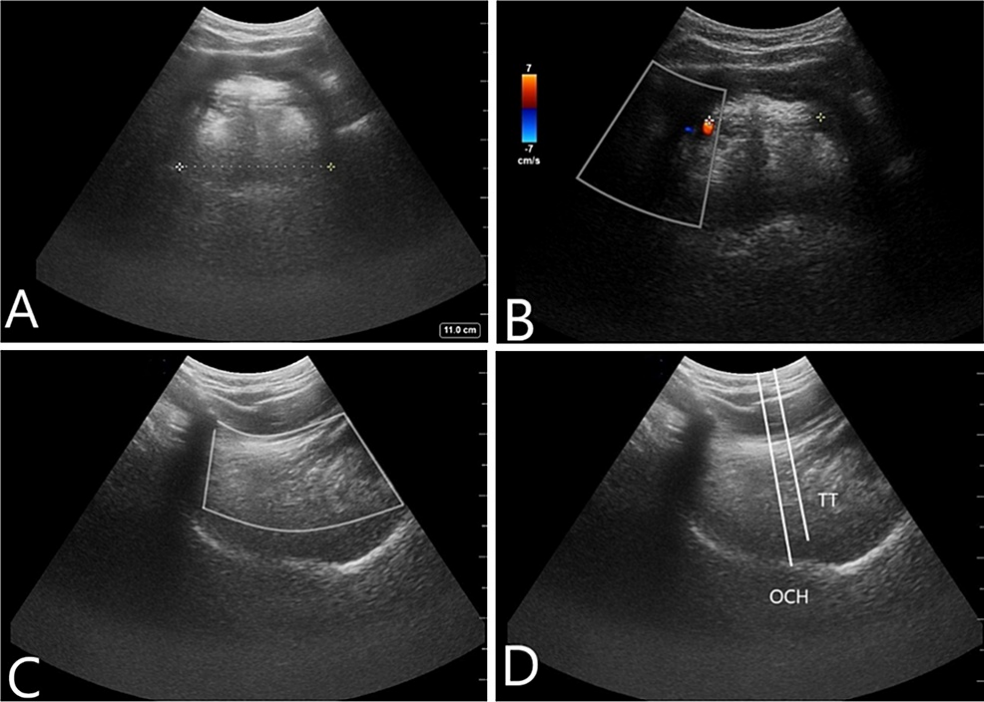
Submandibular ultrasound of the patient’s airway prior to intubation. (A) Tongue width. (B) Distance between lingual arteries. (C) Tongue cross-sectional area. (D) Tongue thickness and oral cavity height. TT: tongue thickness; OCH: oral cavity height.
